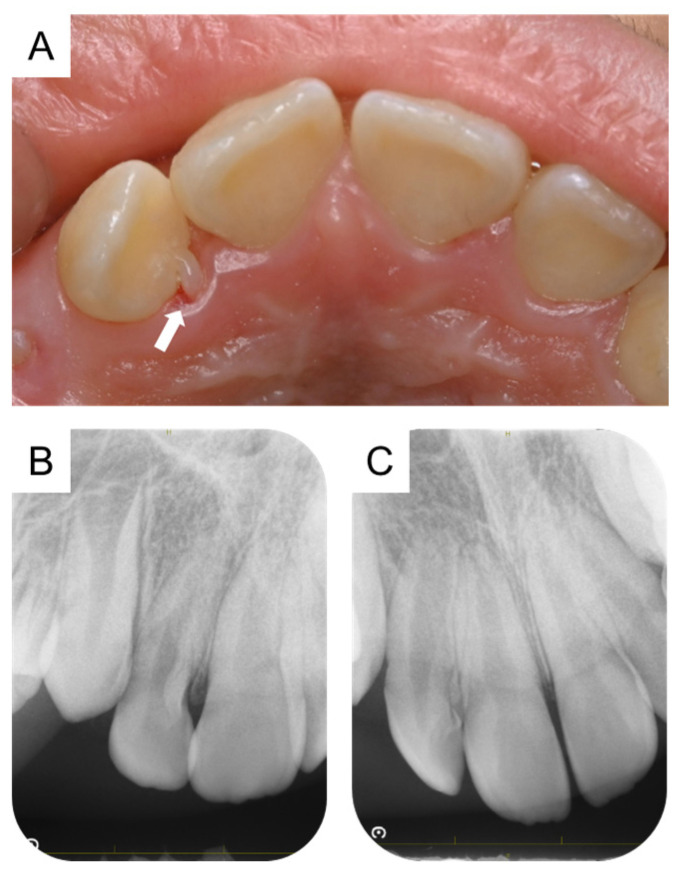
Intraoral photograph and periapical radiograph of maxillary right lateral incisor showing calcified tissue resembling a talon cusp at the age of 11 years and 6 months. (A) Intraoral photograph. Arrows indicate the calcified tissue similar to a talon cusp on the palatal side of the maxillary right lateral incisor. (B,C) Periapical radiographs.
